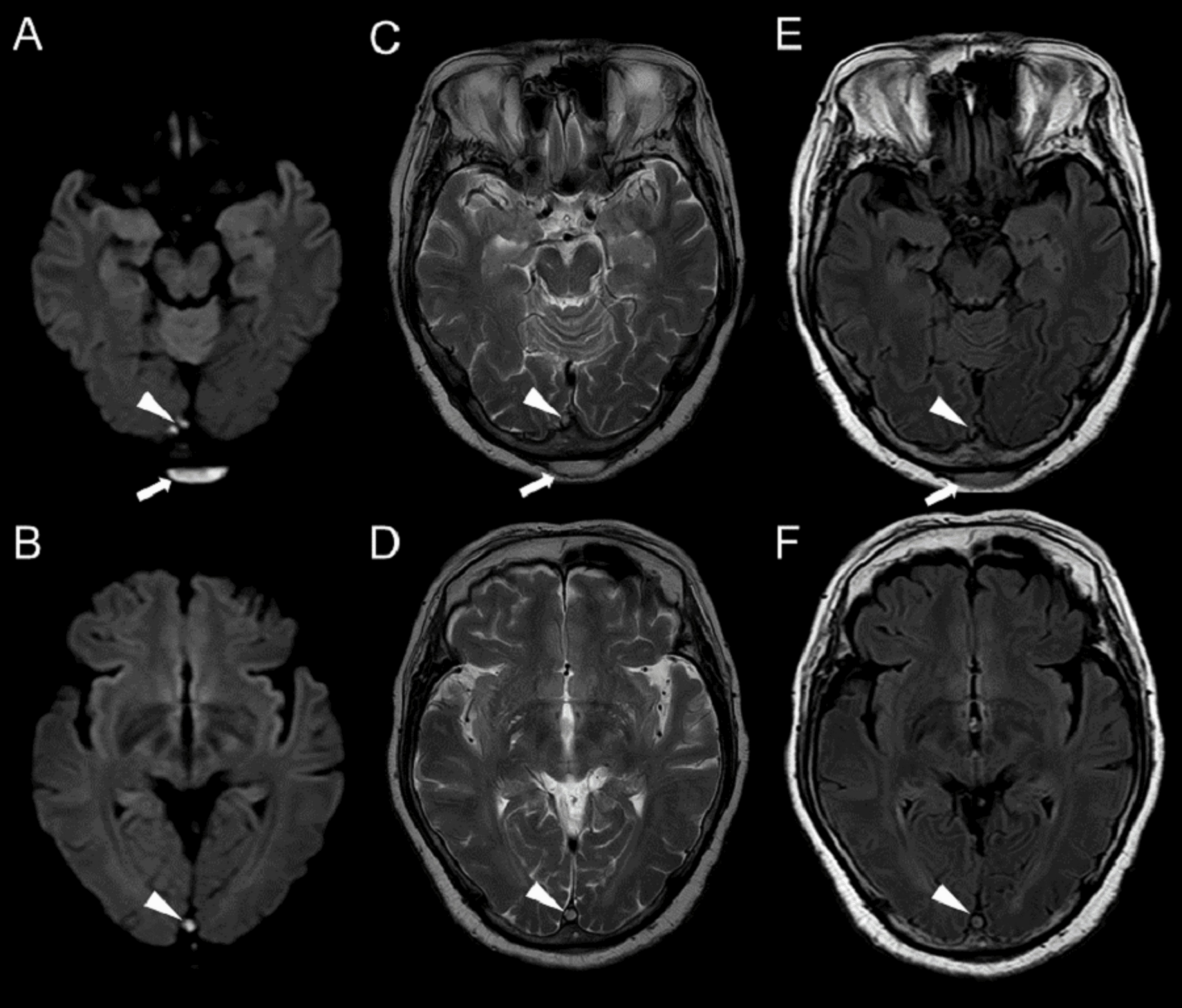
Head MRI A, B: DWI; C, D: T2WI; E, F: FLAIR A 2.4 cm flat fluid collection is observed in the suboccipital region (arrows). At the same level, a high signal is observed in the venous sinus on DWI. Findings are consistent with a suboccipital abscess and venous sinus thrombosis (arrow heads). MRI, magnetic resonance imaging; DWI, diffusion-weighted imaging; T2WI, T2-weighted imaging; FLAIR, fluid-attenuated inversion recovery
